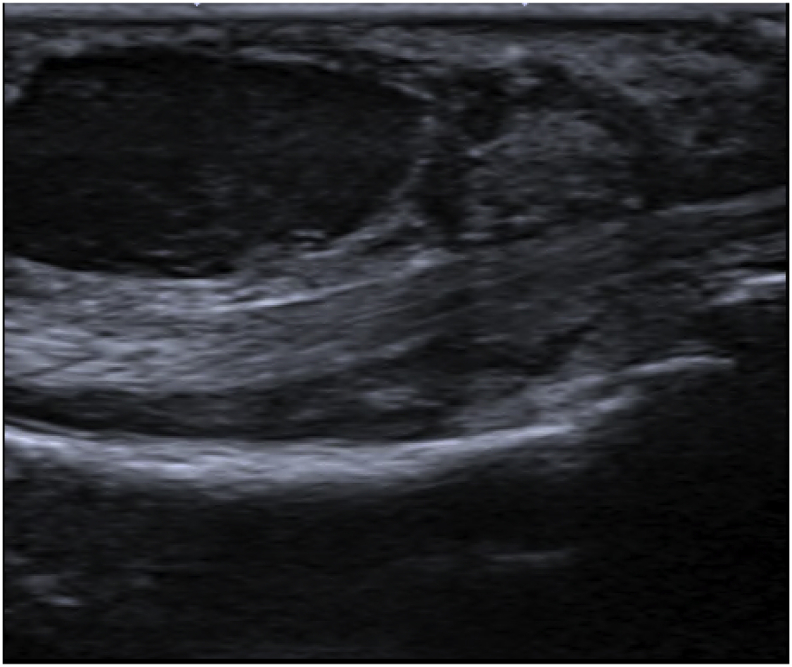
Longitudinal ultrasound view showing hypoechoic with cystic lesion volar to the flexor digitorum tendon of the 5th finger.
